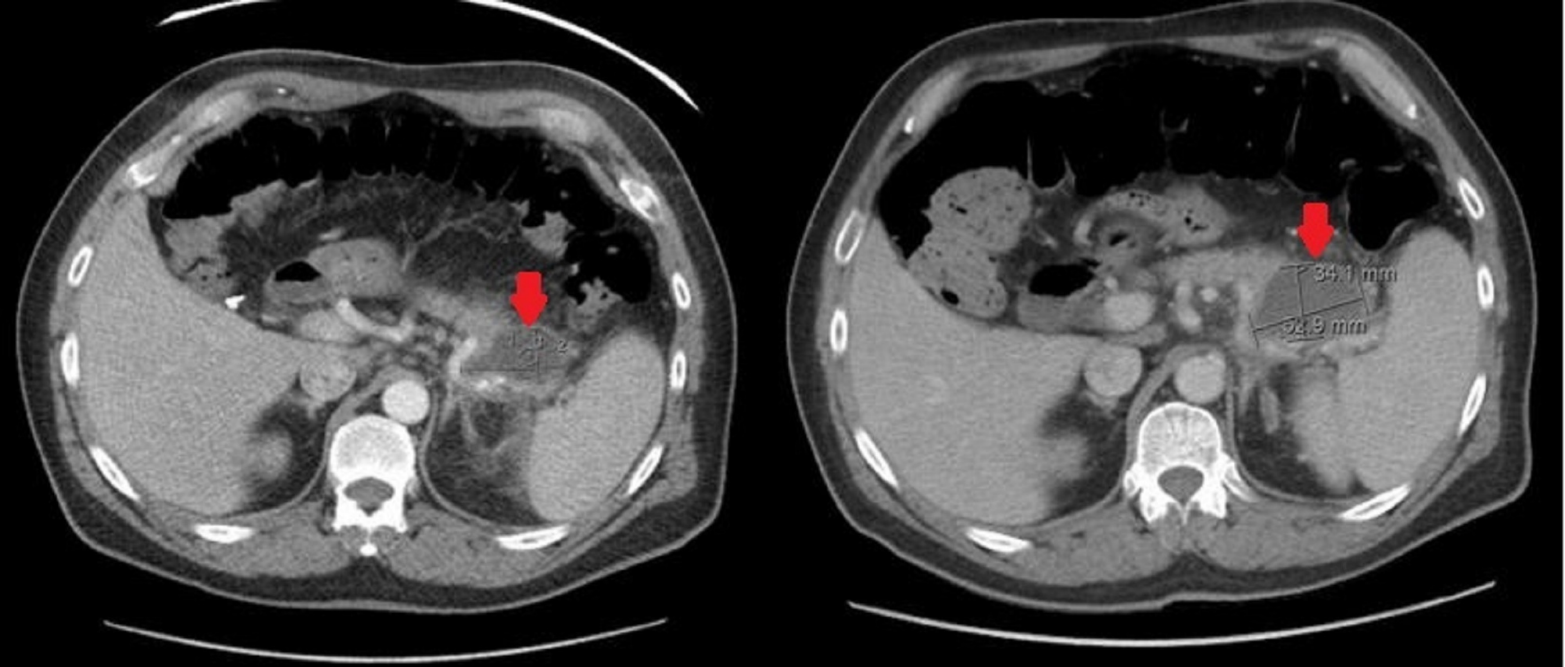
A 5.2 x 2.7 cm cystic lesion was noted on initial computed tomography (CT) abdomen with contrast on prior admission (left, red arrow). Subsequent CT abdomen with contrast two months later (on current admission) showed the lesion had increased to 5.3 x 3.4 cm (right, red arrow).
